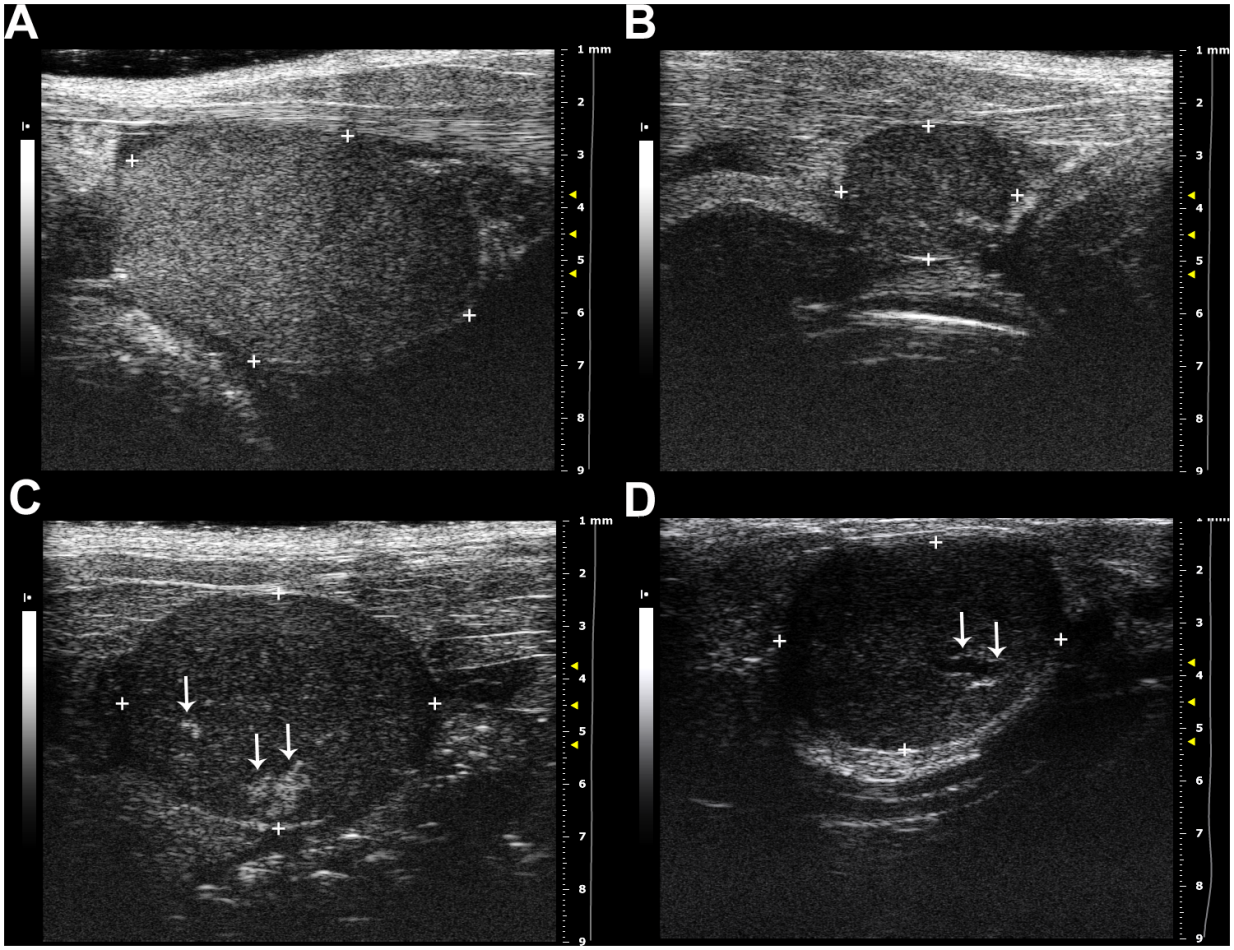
Ultrasonographic appearance of *Fukomys anselli* testicles. Testicles of breeders (A) compared to non-breeders (B) were significantly larger. Irregular hyperechoic lesions were found in 10 out of 13 individuals (C); additionally cystic alterations were present in two out of 13 individuals (D).
